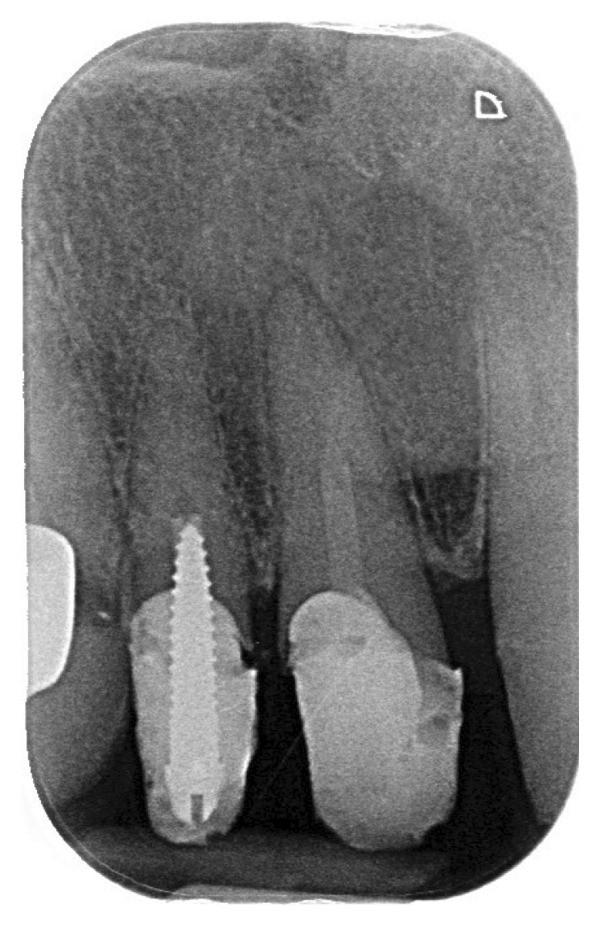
Intraoral radiograph of the central incisors: No periapical radiolucencies are observed.
